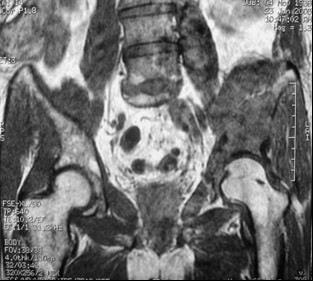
MRI of the corresponding area.
